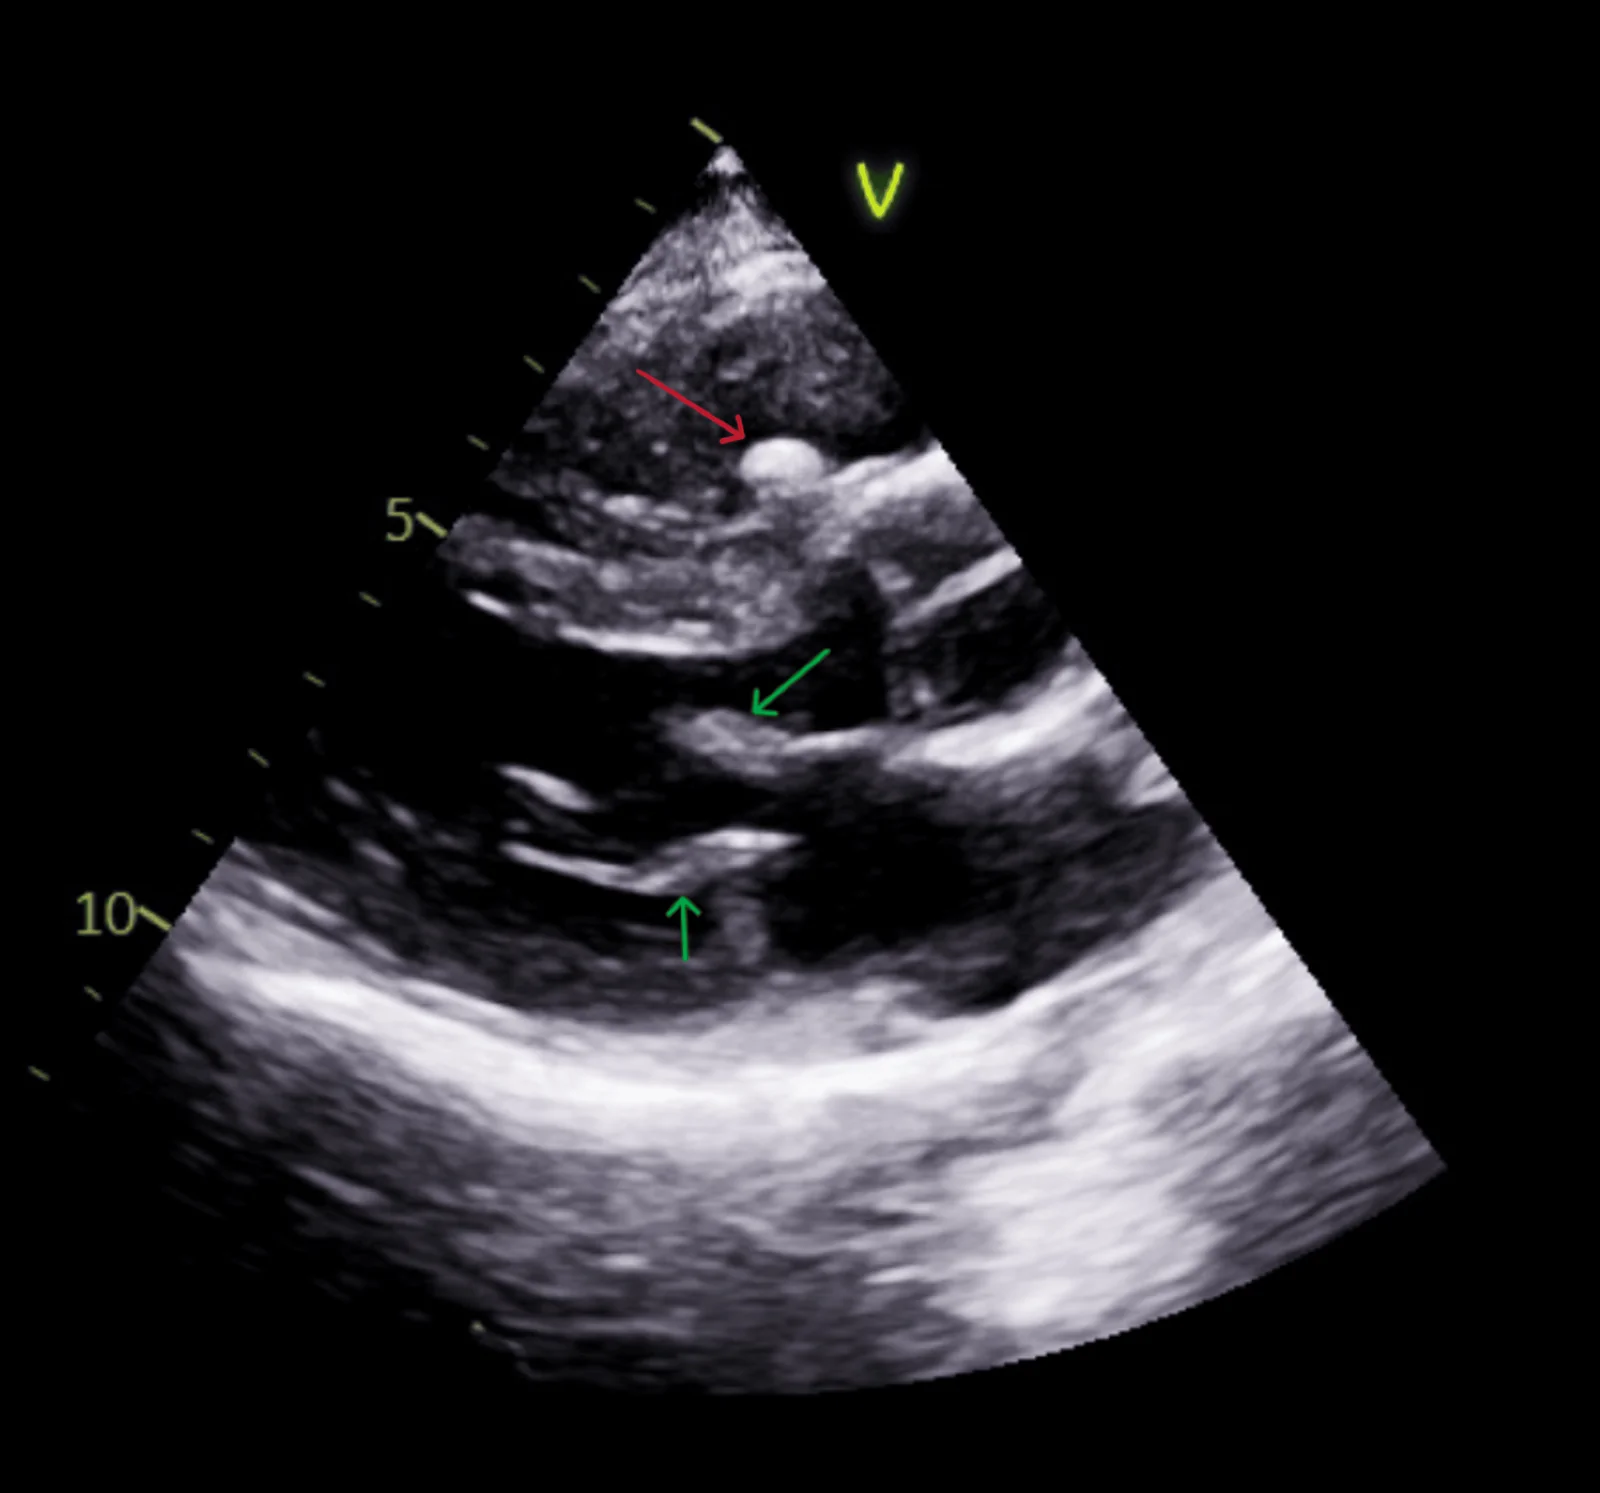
Transthoracic echocardiography demonstrating a mobile echogenic mass present on the mitral valve leaflets (green arrows) and in the right ventricular outflow tract appearing to be attached to the pulmonic valve (red arrow) (parasternal long-axis view).
